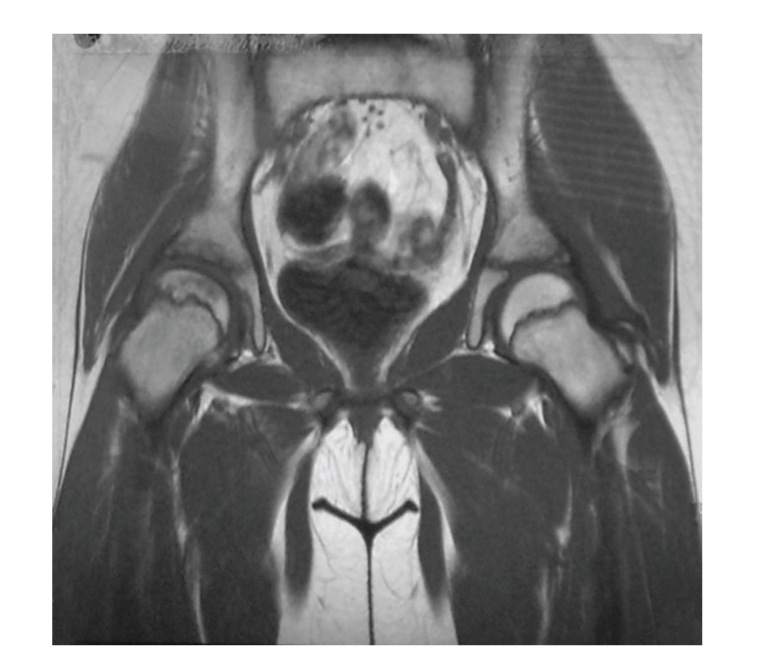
Boundary line of the necrosis of the capital of the femur with a fat-like aspect, good remodelling of the femoral head, and morphologic normalization of the physes are shown in this follow up MRI.
